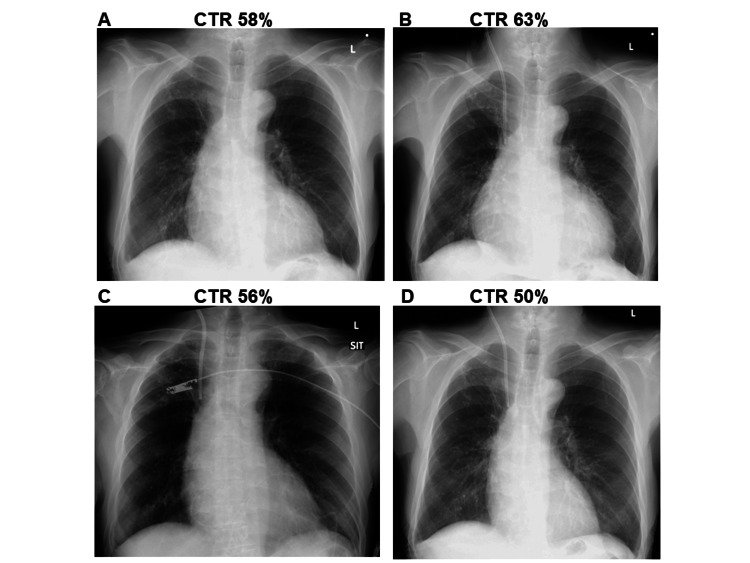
Changes in the cardiothoracic ratio (CTR) on chest radiography. (A) CTR 58% on admission; (B) CTR 63% on the 20th hospital day before pericardiocentesis; (C) CTR 56% on the 21st hospital day after pericardiocentesis; and (D) CTR 50% on the 30th hospital day.
